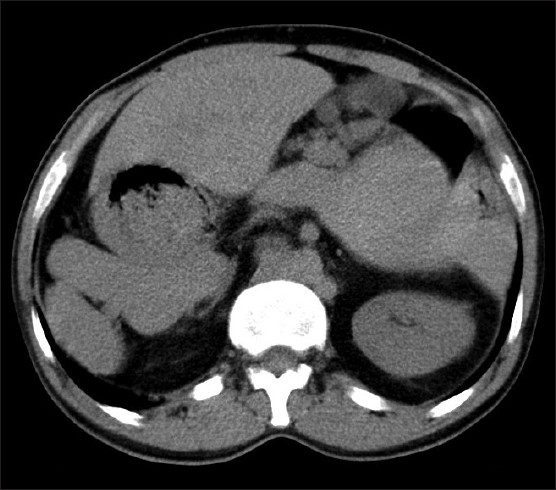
Non-contrast CT of the upper abdomen shows midline liver, stomach on right side of liver and multiple spleens in right hypochondrium.
